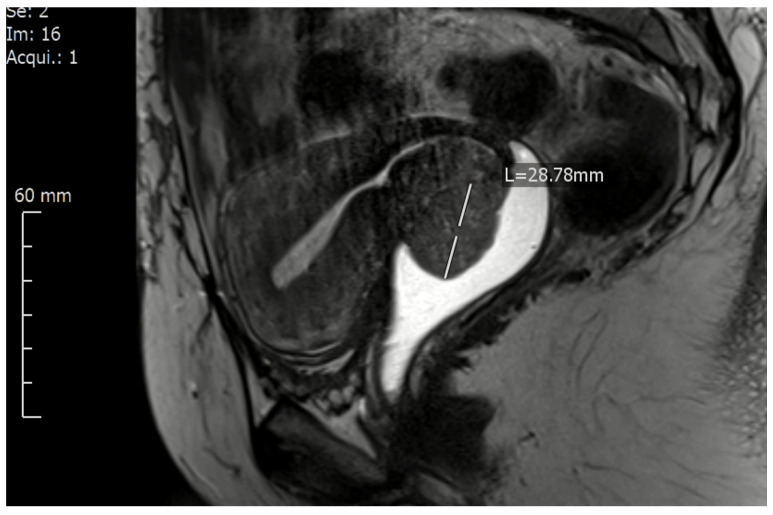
A magnetic resonance imaging of cervical cancer location on the ventral lip of the cervix (FIGO IB2) presented using a contrast agent in the vagina.
